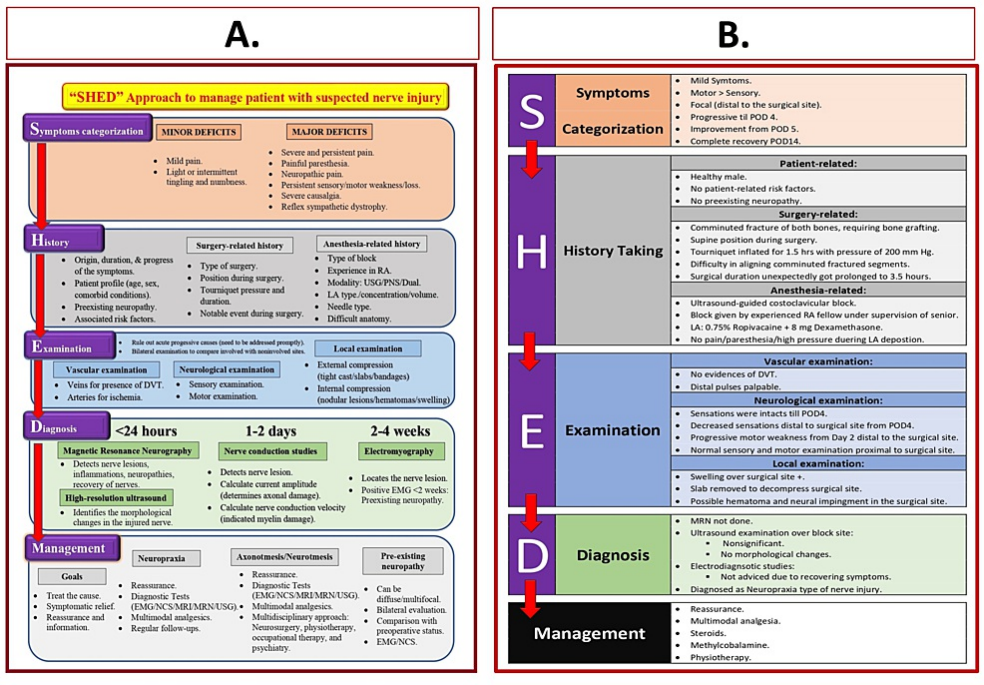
SHED approach to manage perioperative nerve injury. A: Steps of the SHED approach [9]. B: Incorporation of the SHED approach to managing patients with perioperative nerve injury. Source: This figure was created by the first author (KS). RA: regional anesthesia; POD: postoperative day; LA: local anesthetic; USG: ultrasound guidance; PNS: peripheral nerve stimulator; DVT: deep venous thrombosis; EMG: electromyography; NCS: nerve conduction studies; MRI: magnetic resonance imaging; MRN: magnetic resonance neurography
